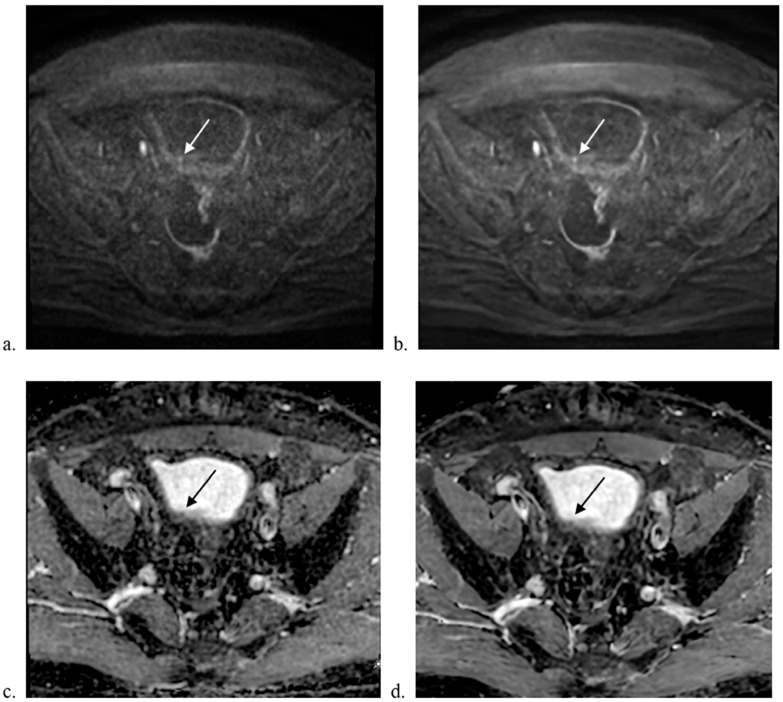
Comparison of MUSE-DWI and DL MUSE-DWI datasets. A 71-year-old male underwent bladder MRI. A tiny papillary lesion was seen at the right posterior wall of the bladder. Transurethral resection confirmed high-grade urothelial carcinoma without involvement of subepithelial connective tissue. Axial images showing (a) MUSE-DWI, (b) DL MUSE-DWI, (c) ADC map generated from MUSE-DWI, and (d) ADC map generated from DL MUSE-DWI. The DL MUSE-DWI dataset showed better lesion conspicuity.
